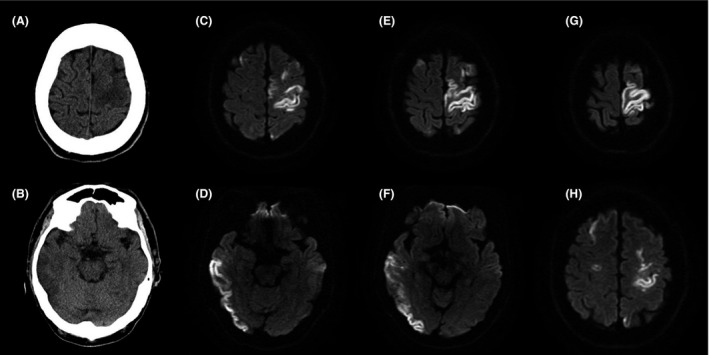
Computed tomography images of a 44‐year‐old man with self‐inflicted stab wounds to the anterior neck and left thorax. Images of the head (A, B) show cerebral infracts in the bifrontal lobe and the right temporal lobe. Magnetic resonance diffusion weighted images (C–H) show multiple high‐intensity lesions along the cortical layer.
